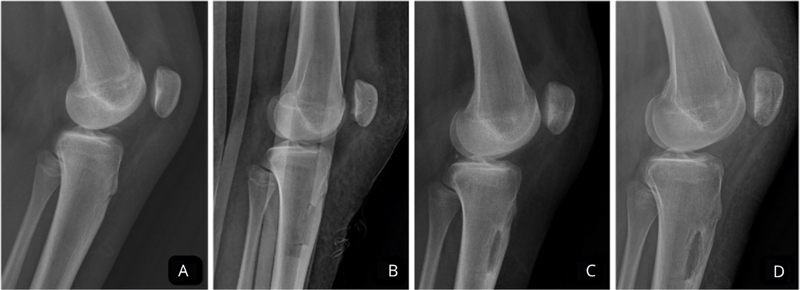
Radiographic progression demonstrating consolidation of a patellar osteochondral lesion.
